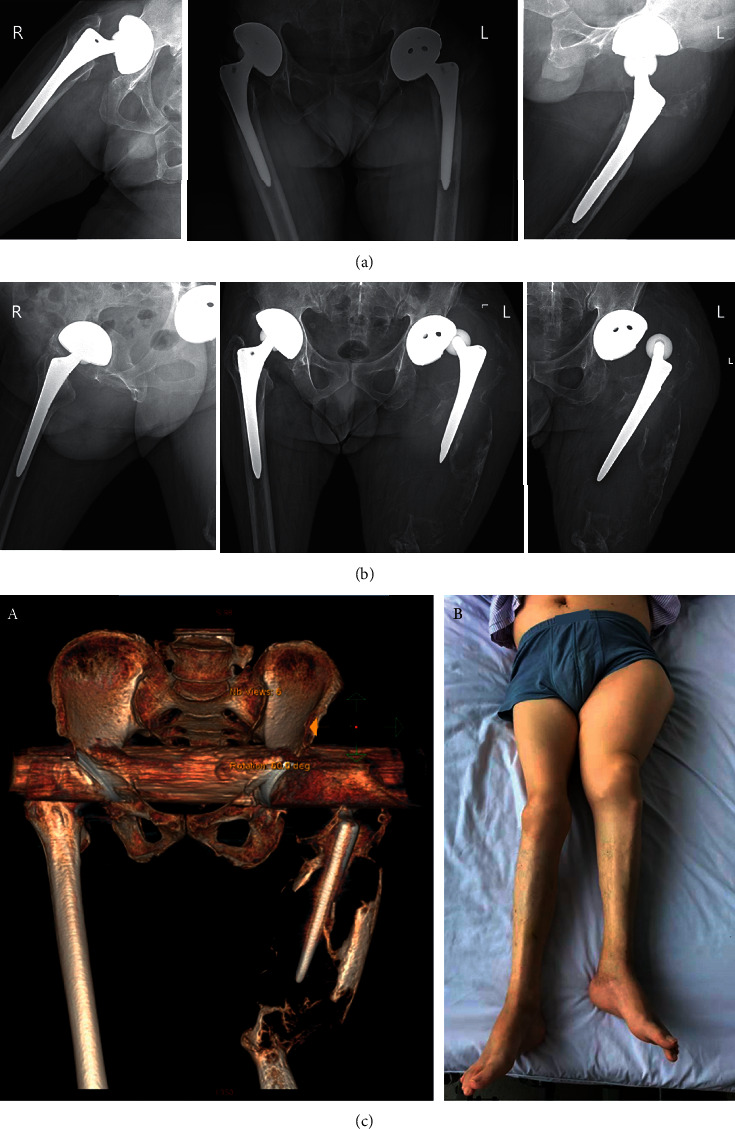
X-ray photography of hip joints of Patient 1. (a) Taken two months after trauma. (b) Taken eight months after trauma, nearly the entire left femur displayed a characteristic “soap bubble” appearance, and the femoral stem “floated” in a large pseudotumor. (c) CT reconstruction (A) and general photography (B) taken eight months after trauma.
